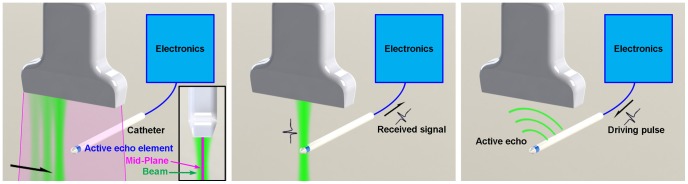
The configuration of the active reflector tracking system. Left: the imaging probe fires a serious of ultrasound beams scanning the image region. The center plane of the image region is the mid-plane. Middle: When an ultrasound beam intersects the active echo element, an electrical pulse is received by the electronics. Right: The received pulse triggers the electronics, a driving pulse is sent to the element to fire an active echo pulse.
